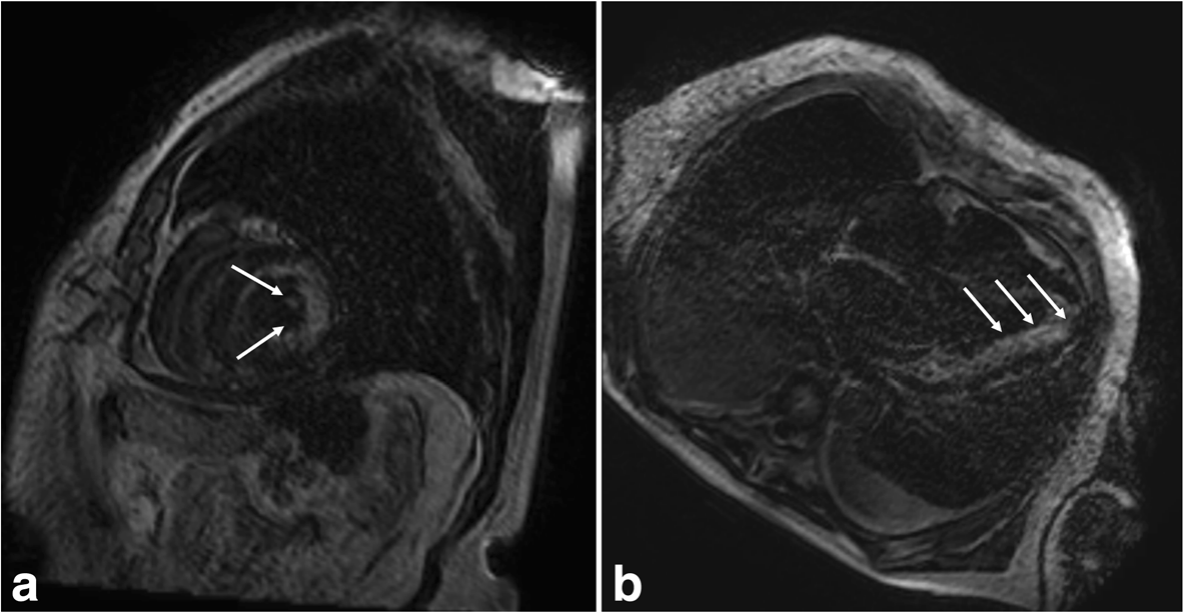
a and b Cardiac magnetic resonance imaging short-axis (a) and four-chamber (b) late gadolinium enhancement images showing diffuse subendocardial scar (*arrows*) at the lateral wall of the left ventricle, a finding consistent with subendocardial infarction subsequent to chronic subendocardial ischemia
